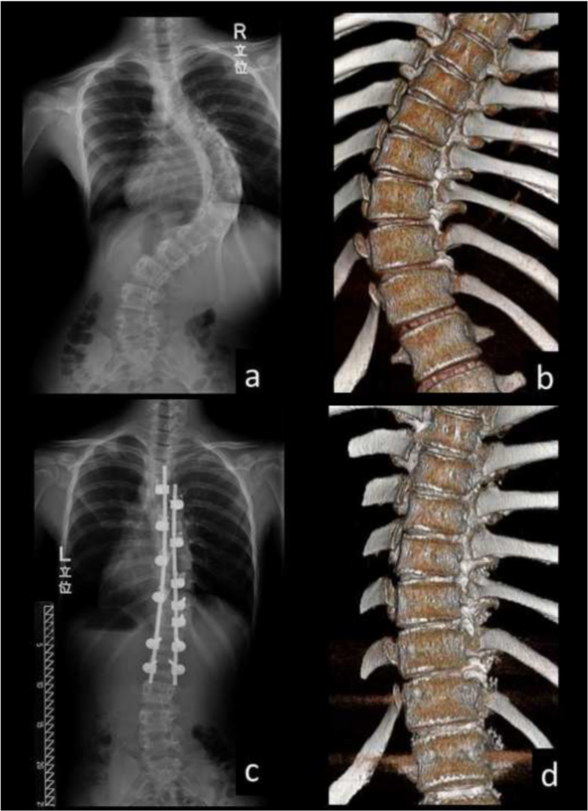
Preoperative (a, b) and postoperative (c, d: 1 week postoperatively) full-length standing posteroanterior radiographs and 3D reconstructed CT images in the main thoracic curve of a 12-year-old female patient (Patient No. 5 in Table 1)
